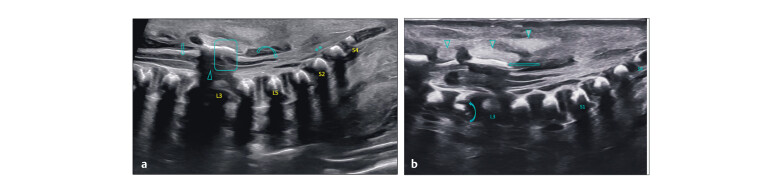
Postmortem ultrasound: (
